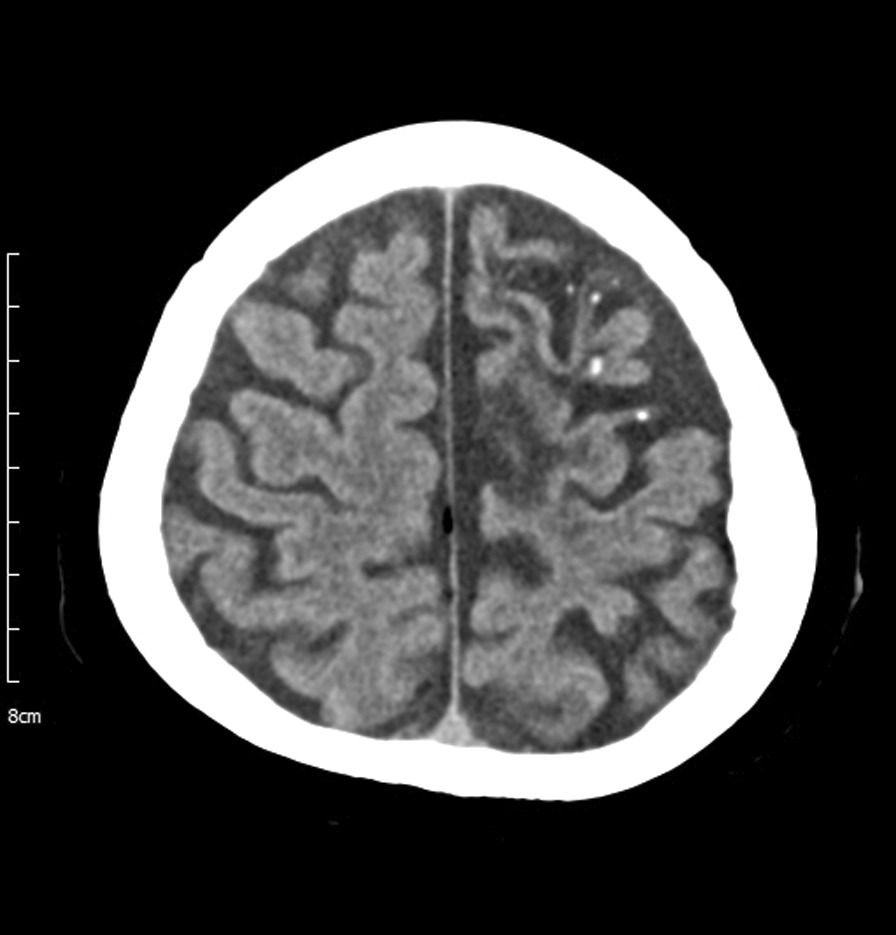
Brain computed tomography of the patient
